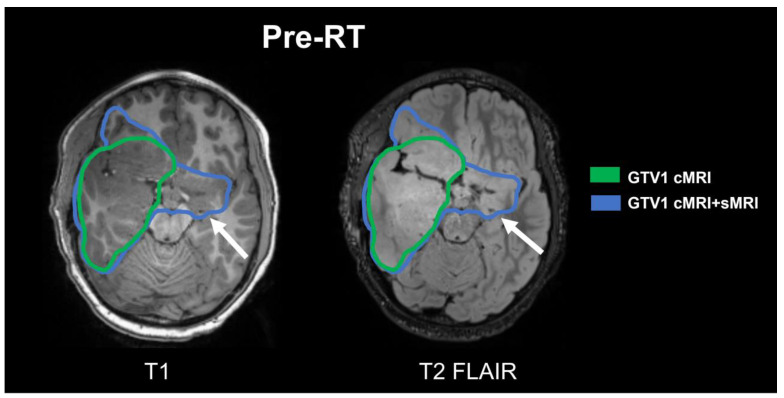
Comparison of GTV1 contours. Target volumes based on cMRI alone (green) and cMRI + sMRI (blue) are shown overlaid on axial slices of the T1 (left) and T2 FLAIR (right) images. The sMRI detected an additional lesion in the left temporal lobe which is non-hyperintense on FLAIR and absent on the T1 imaging as well (white arrow). The volume of GTV1 cMRI was 207.61 cc compared to the GTV1 cMRI + sMRI volume of 297.84 cc.
